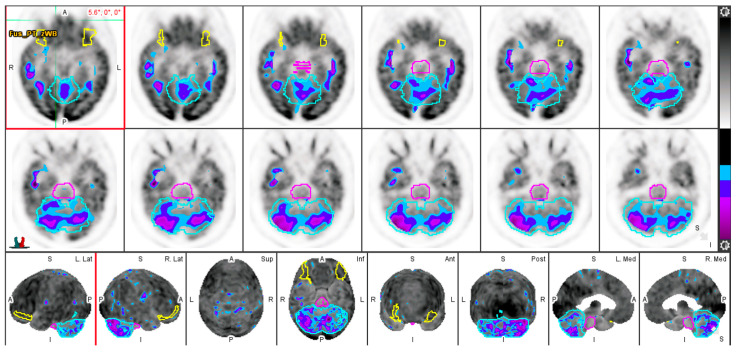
Evaluation of FDG-PET through quantitative analysis. Low FDG uptake is represented by purple and blue contours. The basis pontis (pink), cerebellum (light blue), and lateral orbital gyrus (yellow) are delineated.
